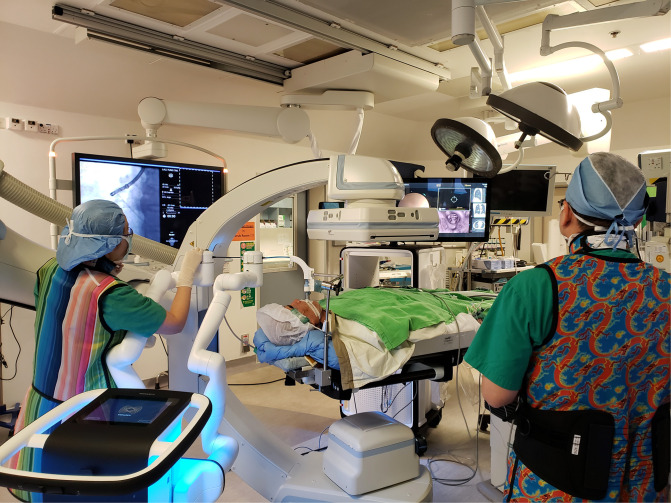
Patient lies supine, intubated by single-lumen endotracheal tube under general anaesthesia. The robotic bronchoscope enters the endotracheal tube *via* the head-side. Robotic bronchoscopy guided by CBCT in our hybrid operating room is usually performed by two operators. One operator (right) performs fine-tuning of the direction of bronchoscope using the controller console, another operator (left) controls the advancement of instruments and commands fluoroscopic screening using different angles of C-arm. The lesion is usually marked on the fluoroscopy screen using i-guide® CBCT platform so that the relationship between instruments and lesion can be seen on live fluoroscopy. In this figure, the robotic arm can be seen in very close proximity with the C-arm. Both the robotic platform monitor and the fluoroscopy screen should be visible to both operators, and communication is important for coordination.
